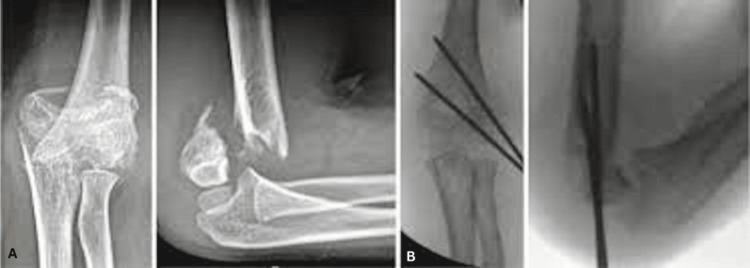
Divergent lateral pinning A: Preoperative anteroposterior and lateral radiographs of a 10-year-old boy; B: Immediate postoperative radiograph with good reduction
